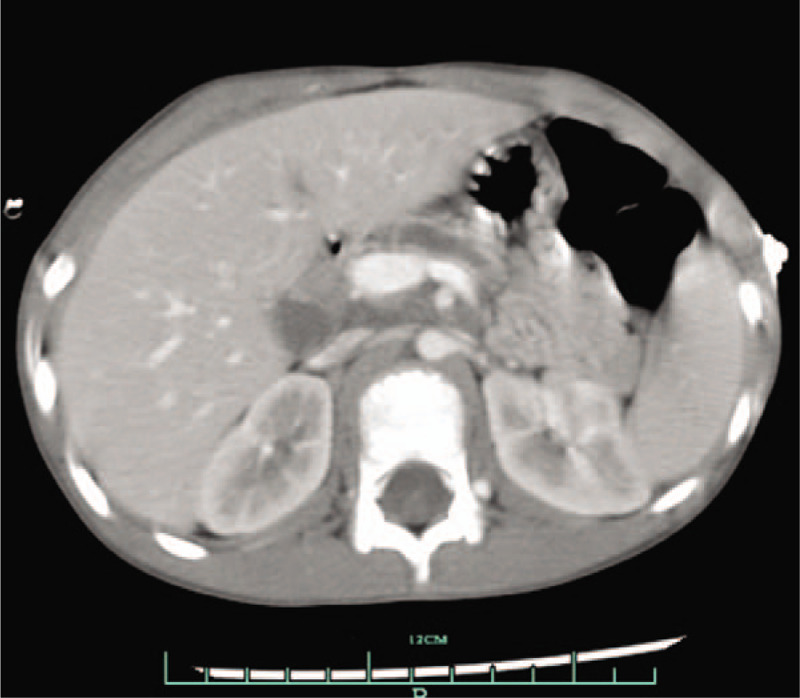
Abdominal CT showed dilated irregular pancreatic duct. CT = computed tomography.
